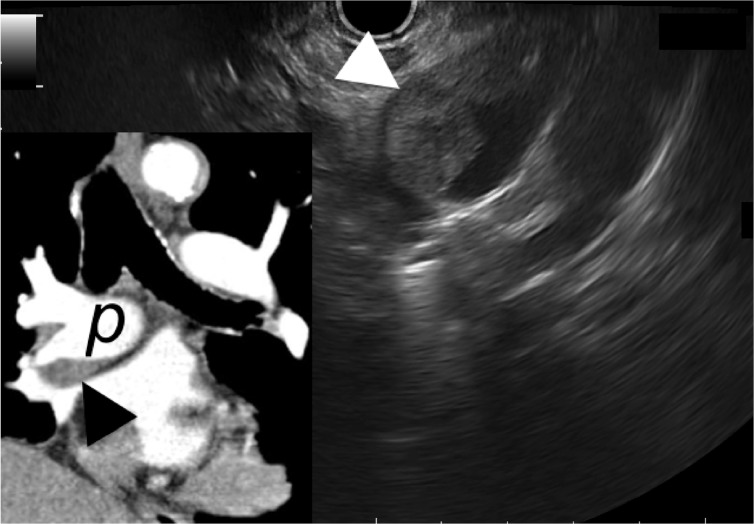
Endoscopic ultrasound image of a heterogeneous hypoechoic intraluminal lesion of the right pulmonary artery (arrowhead). The endoscope is advanced at the mid-oesophagus and orientated towards the anterior and right lateral wall of the oesophagus. Computer tomography scan showed an invasion of the right pulmonary artery (p) by the tumour (arrowhead).
